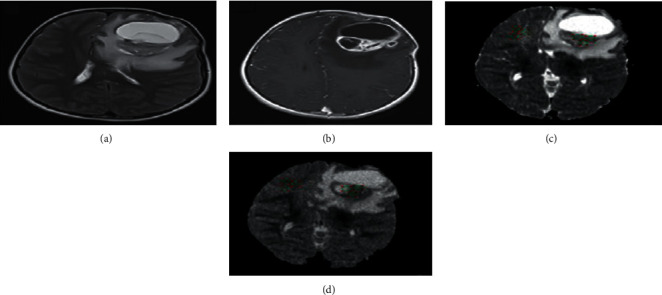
12-year-old female patient with astroblastoma (grade II) in the left frontoparietal lobe. Based on the signal characteristics of T2WI and postcontrast T1WI, three ROIs were placed on the solid component of the tumor and three ROIs were placed on the contralateral NAWM on ADC images. Images (c, d). (a). On the T2 FLAIR sequence (TR/TE = 8800/120 ms), the tumor is hyperintense. (b). On T1WI SE (TR/TE = 410/9 ms) after contrast injection, the tumor is hypointense with ring enhancement. (c). ADC map at a standard *b* value (TR/TE = 6100/152 ms; *b* = 1000s/mm^2^). (d). ADC map at a high *b* value (TR/TE = 8600/152 ms; *b* = 3000s/mm^2^). The tumor is more hypointense on the ADC map obtained at a high *b* value than the ADC map obtained at a standard *b* value.
